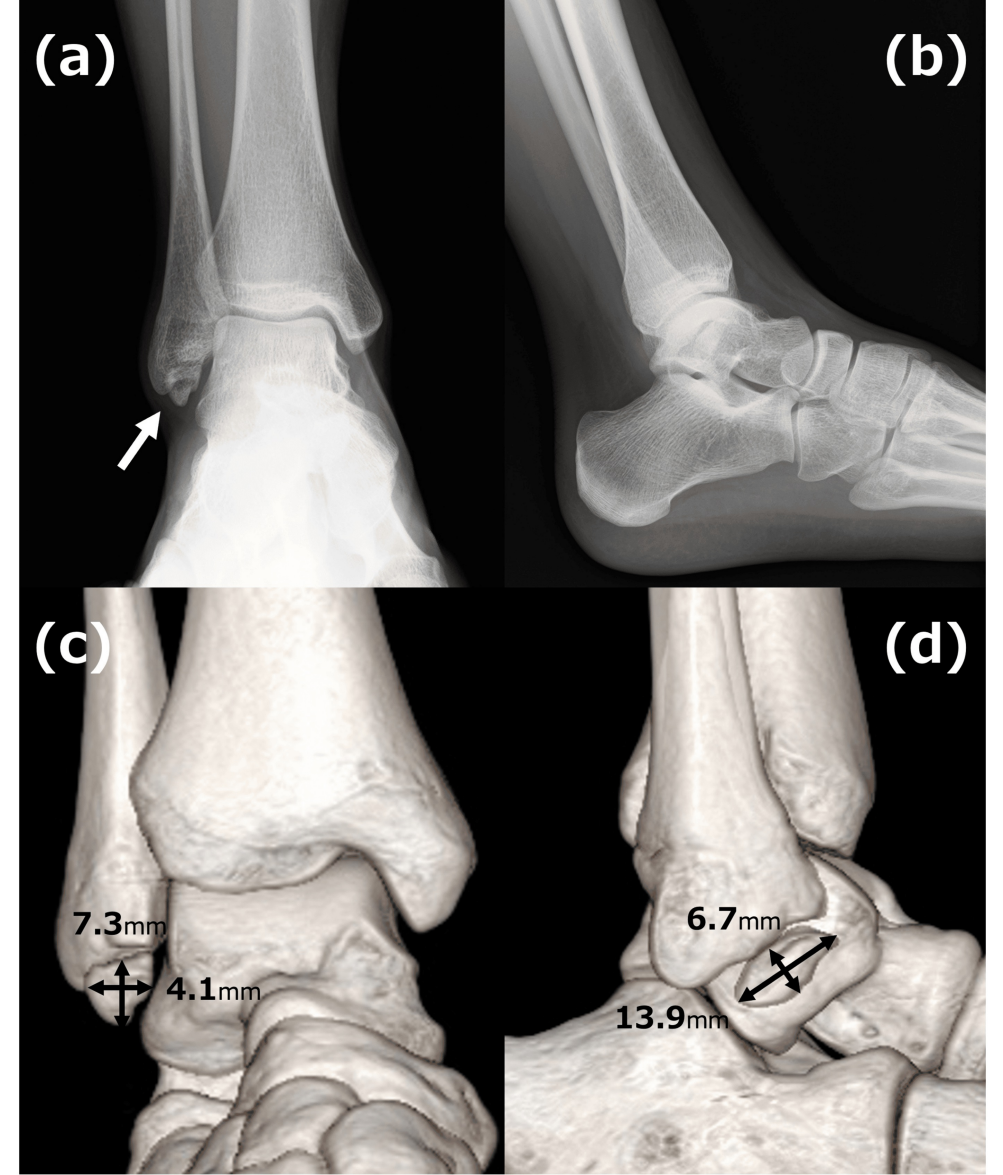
Radiographic and 3D CT imaging of the ankle. Plain radiographs: Anteroposterior view (a) and lateral view (b). An arrow identifies the os subfibulare. 3D reconstructed CT images: Anterior view (c) and lateral view (d). The os subfibulare measures 14 mm in maximum diameter.
